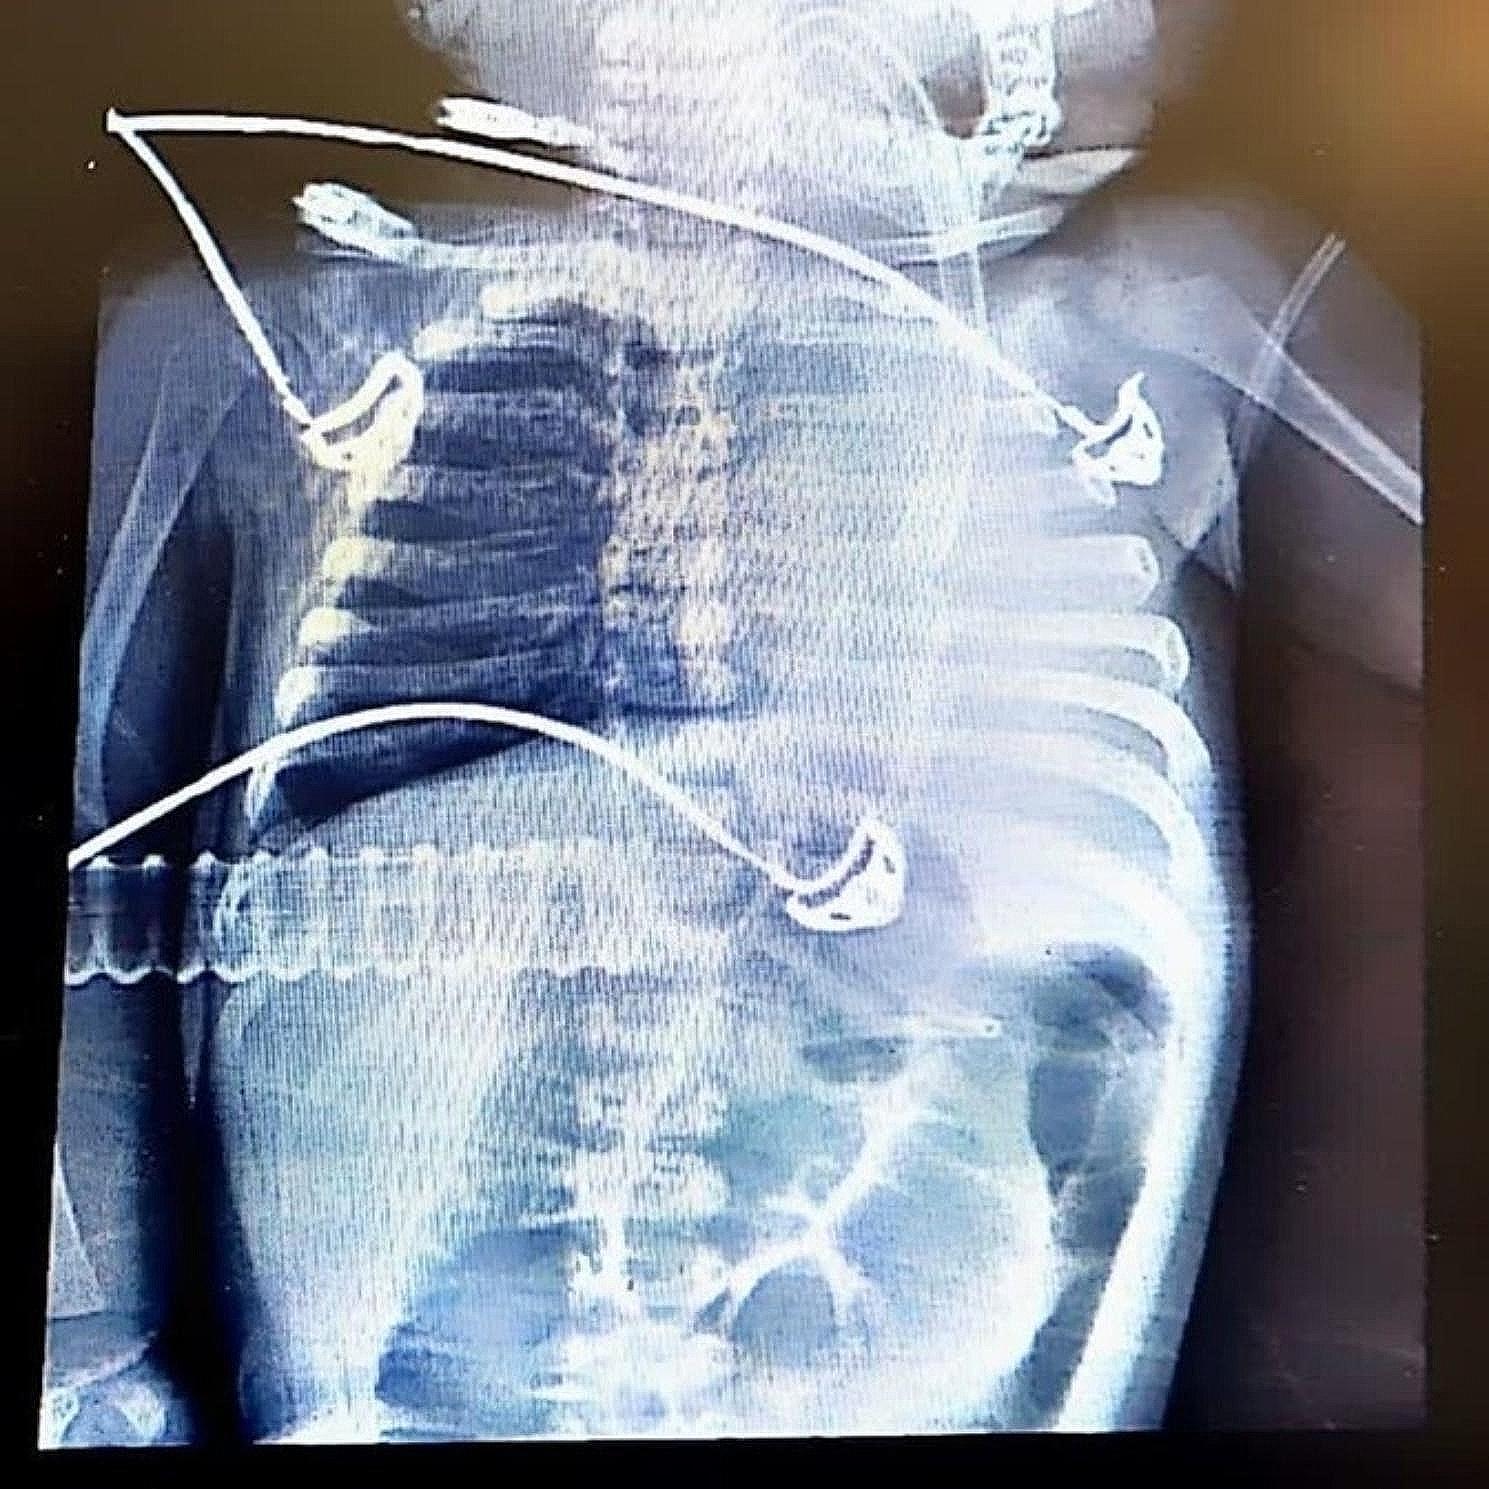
Admission Chest X-ray demonstrating cardiomegaly
